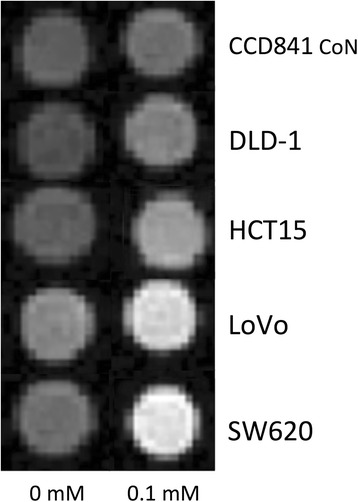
In vitro manganese-enhanced magnetic resonance imaging of cell lines. T1-weighted imaging of cell pellets incubated with and without 0.1-mM MnCl_2_. Cancer cells enhance more markedly than do normal cells and high-metastatic-potential cells SW620 and LoVo enhance the most
